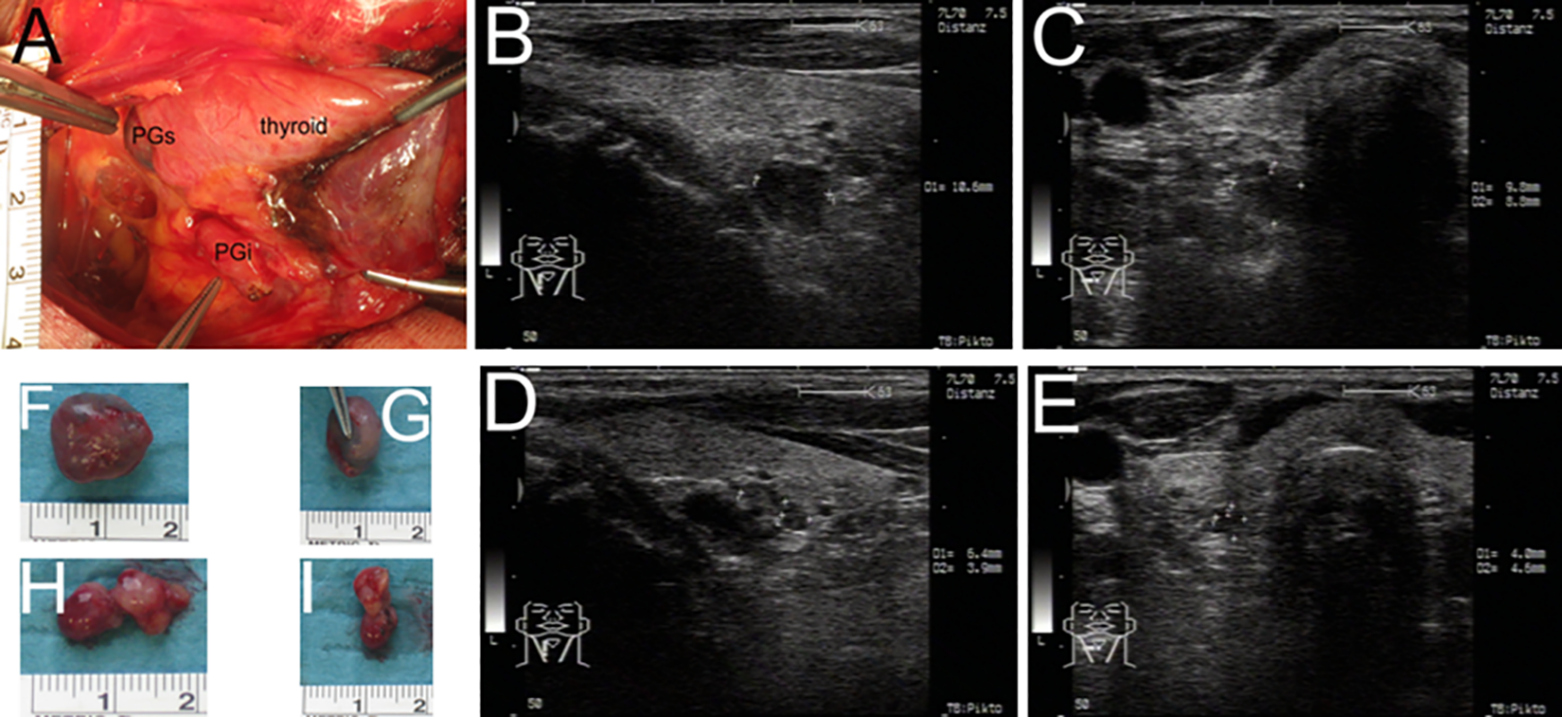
Topography and size of parathyroid glands at parathyroidectomy and ultrasound. Topography of the right-sided superior and inferior PG after luxation of the thyroid gland (A). Topography and measurement of corresponding PG by US: superior (B+C) and inferior (D+E). Volumes of PG were 479mm^3^ (PG superior) and 99mm^3^ (PG inferior) when calculated with US measurements. Documentation of PG size during surgery: superior (F+G) and inferior (H+I). Volumes of PG were 858mm^3^ (PG superior) and 293mm^3^ (PG inferior) when calculated with intra-operative measurements. PGs, superior parathyroid gland; PGi, inferior parathyroid gland.
